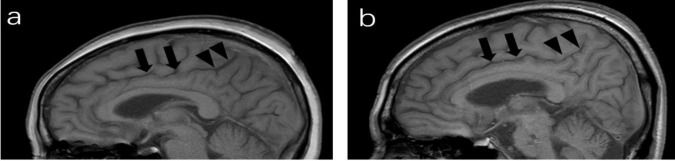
Paramedian sagittal T1-weighted image of MRI; (a) a 64-year-old man (negative), the posterior part (arrowheads) of the cingulate sulcus is slightly wider than the anterior part (arrows). (b) cingulate sulcus sign (CSS): a 67-year-old man, the anterior part of the cingulate sulcus are wide (arrows), whereas the posterior part is tight (arrowheads).
